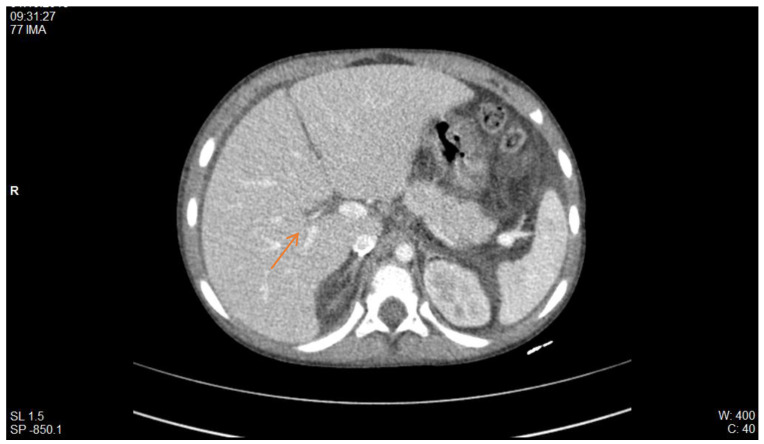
CT scan: thrombosis of the inferior vena cava with extension to the right renal vein (red arrow) (personal collection Nephrology Department).
